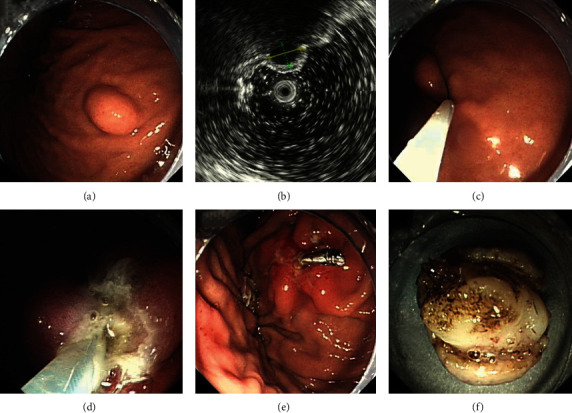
Endoscopic snare resection treatment for a gastric GIST: (a) gastric GIST identified by endoscopy; (b) the same gastric GIST observed by endoscopic ultrasound; (c) encirclement of the tumor by snare; (d) resection of the tumor; (e) close the wound; (f) the resected tumor. GIST: gastrointestinal stromal tumor.
